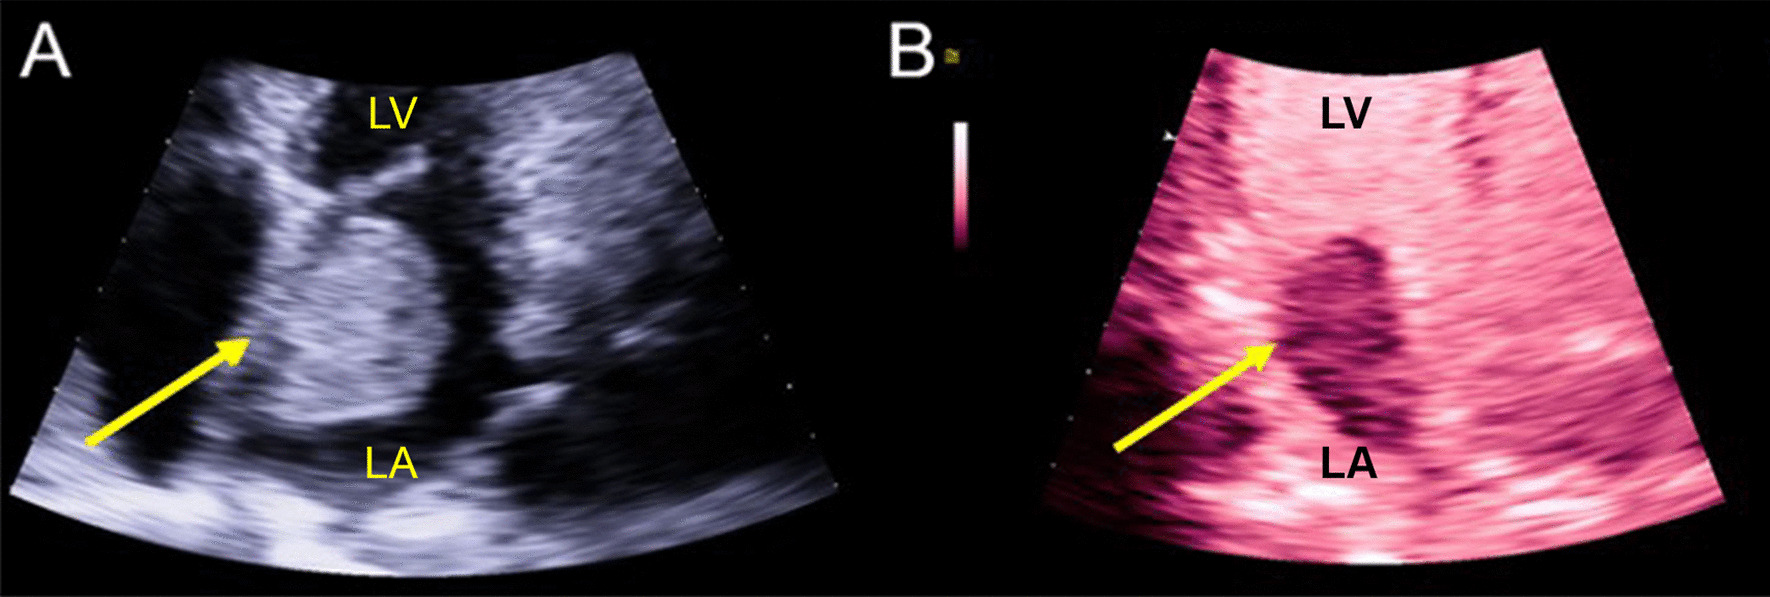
Myxoma as a Heterogeneous Hyperechoic Mass with Definity Enhanced Imaging. a Echocardiographic image showing (3 cm × 4.8 cm) heterogeneous hyperechoic mass in left atrium attached to the interatrial septum by a stalk (arrow). b Definity enhanced ultrasound of left atrial myxoma demonstrating heterogeneous echogenicity within the mass representative of vascularity
